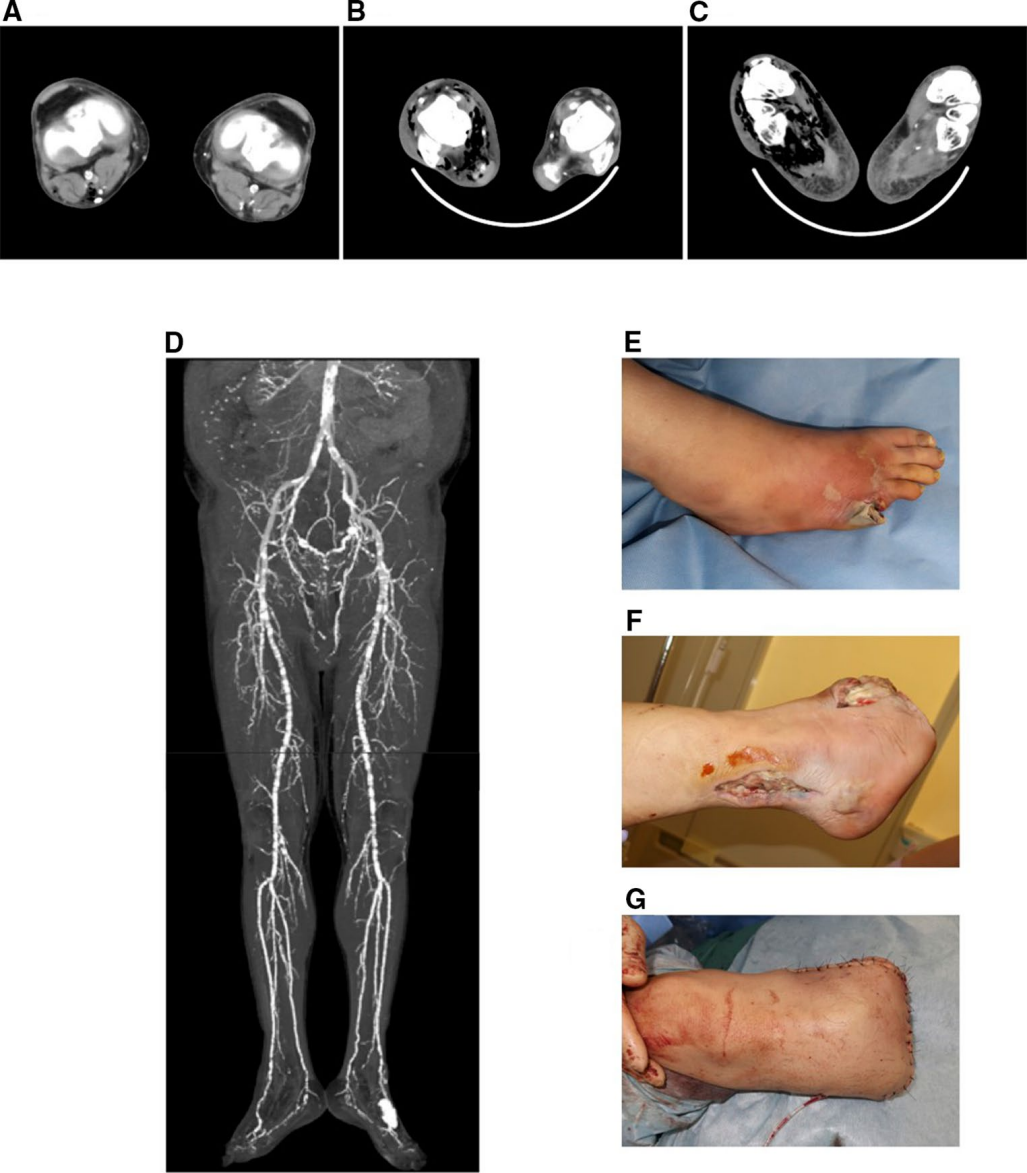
a–c Leg computed tomography (CT) showing subcutaneous extensive foci of gas throughout his whole leg region. a Knee joint region, b lower leg region, c toe region, d CT angiography showing severe arterial calcification throughout the whole body, e–g non-clostridial gas gangrene in right foot, e after amputation of his right little toe, f after amputation of his right foot from Lisfranc’s joint, g after amputation of his right foot at upper 15 cm of medial condyle
